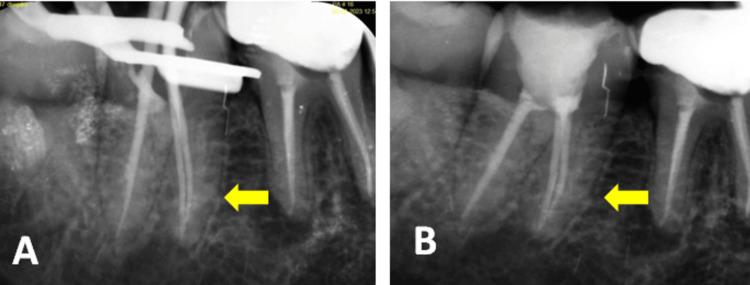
Intraoral periapical radiographs of 47 (A) Master cone verification and (B) postoperative radiograph
